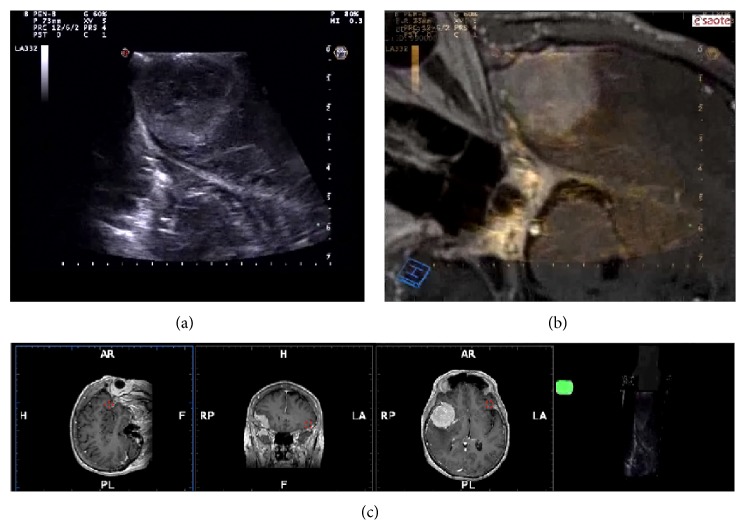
US system screenshot. In the upper panel on the left a standard real-time ioUS B-mode image is displayed; on the right, the corresponding preoperative MRI is fused with real-time ioUS B-mode. In the lower the three standard orthogonal planes (sagittal, coronal, and axial) and the insonation plane panel are displayed.
